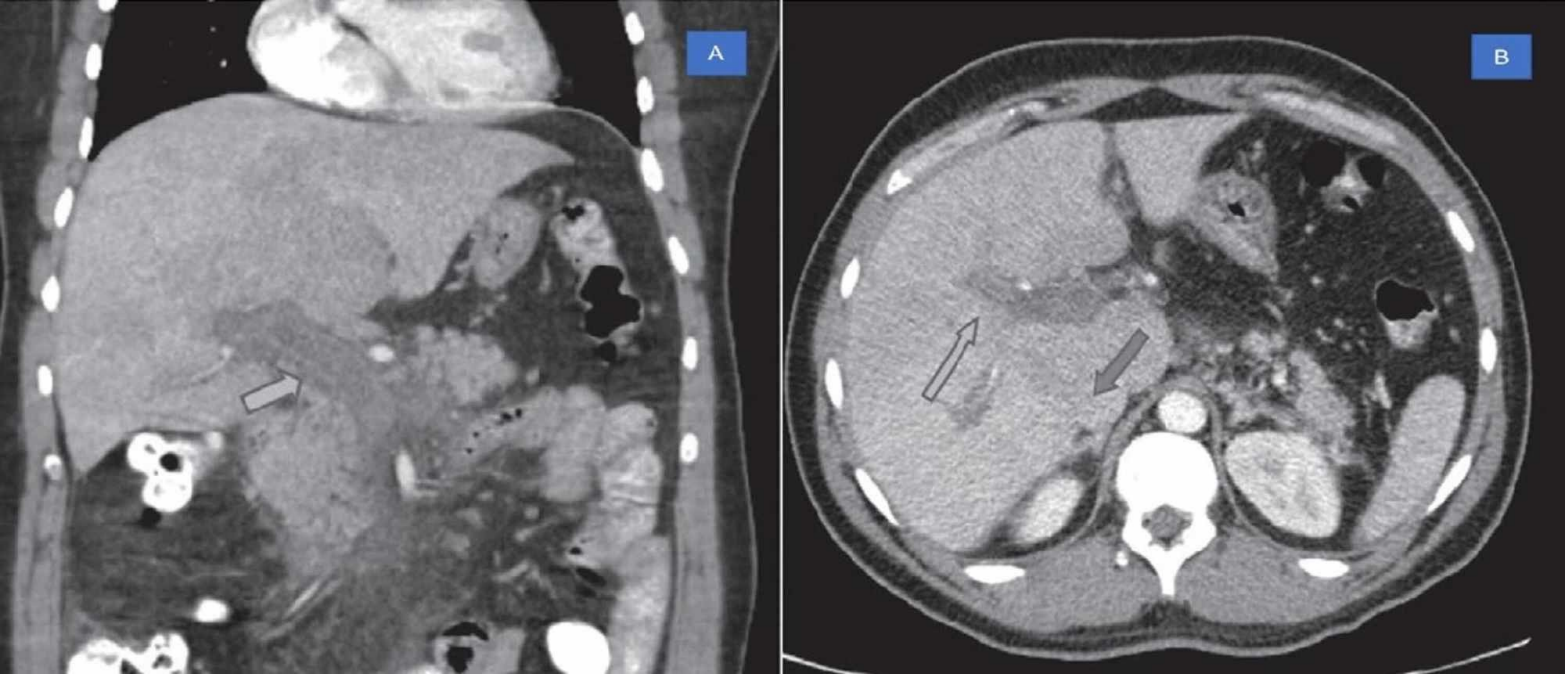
CT scan A: CT demonstrating filling defect within the portal vein representing portal vein thrombosis (PVT) (shown by arrow). B: PVT in the main portal vein and left portal vein (shown by arrows).
